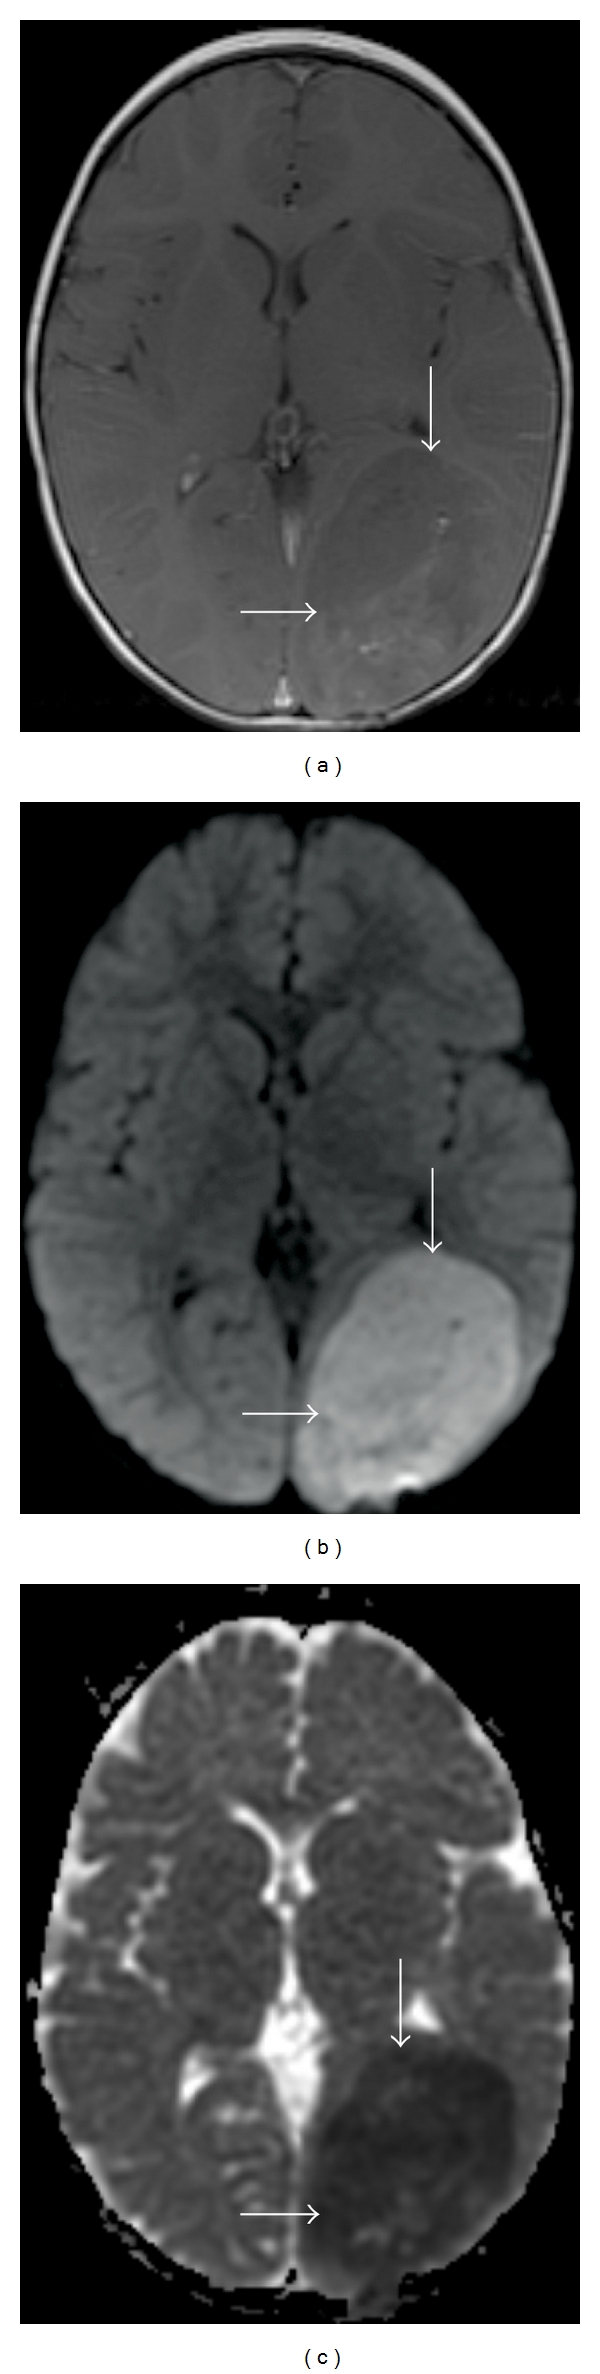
A 2-year-old female with a left parietal primitive neuroectodermal (PNET), WHO grade IV. (a) Axial T1 postcontrast. A faintly enhancing left parietal lobe mass is present (arrows). (b) DWI sequence. The mass is hyperintense on diffusion-weighted imaging. (c) ADC map. Signal hypointensity of the lesion on the ADC map (arrow) is present, consistent with restricted diffusion related to dense cell packing.
